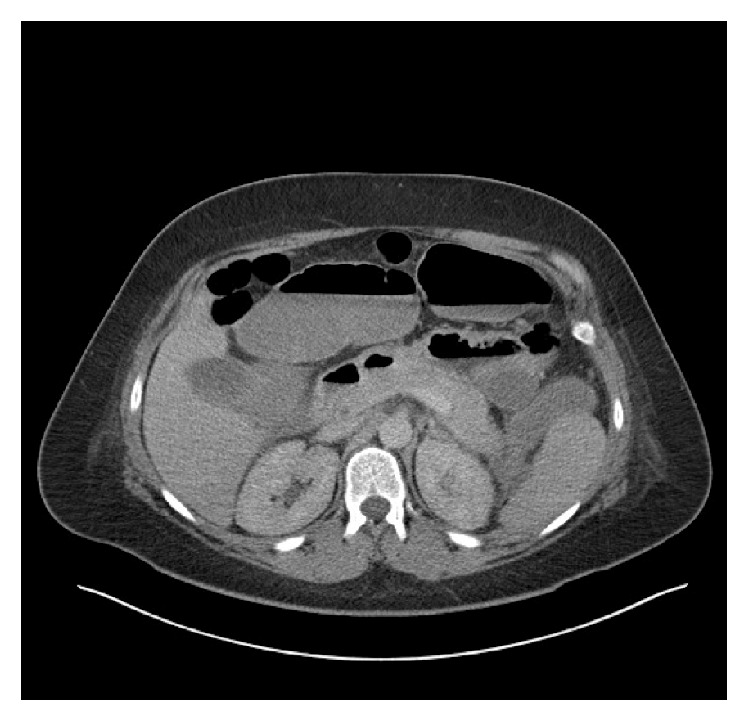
CT scan showing acute pancreatitis and bowel dilatation.
